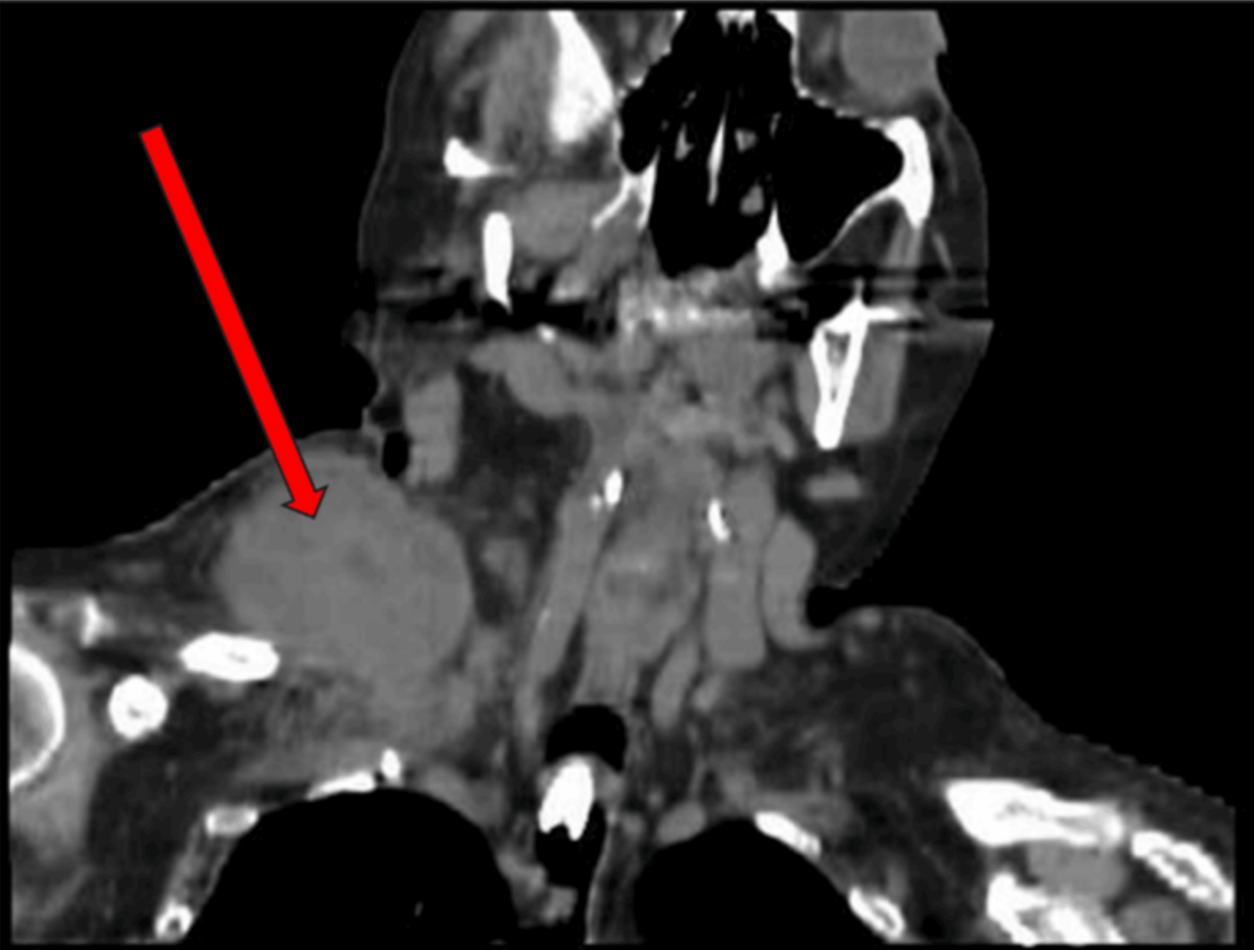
CT neck without contrast of right supraclavicular mass The red arrow depicts a significant interval increase of the right supraclavicular mass, most concerning for a primary neoplasm or metastatic disease, now measuring 7.1 x 6.1 x 8.0 cm. The mass extends below the clavicle approximately 3 cm. The mass also appears to invade the posterior aspect of the right sternocleidomastoid muscle.
